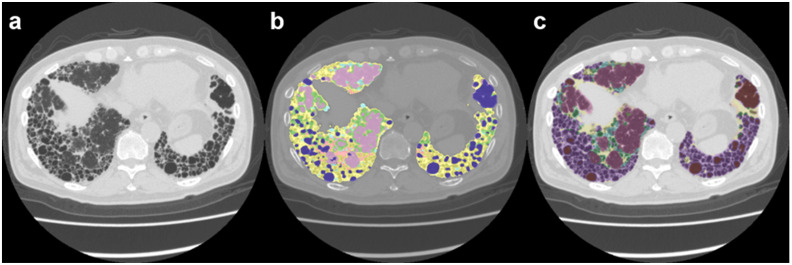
Images of a 72-year-old man with idiopathic pulmonary fibrosis (IPF). (a) Computed tomography image; (b) computer-aided diagnosis (CAD) method image; (c) deep-learning (DL)-based analysis image. Note: For the CAD image, purple = N_CAD_; light green = G_CAD_; pink = C_CAD_; light blue = R_CAD_; yellow = H_CAD_; and dark blue = E_CAD_. For the DL-based analysis image, violet = N_DL_; light green = G_DL_; yellow = CF_DL_; light blue = R_DL_; dark green = T_DL_; purple = H_DL_; and brown = E_DL_.
